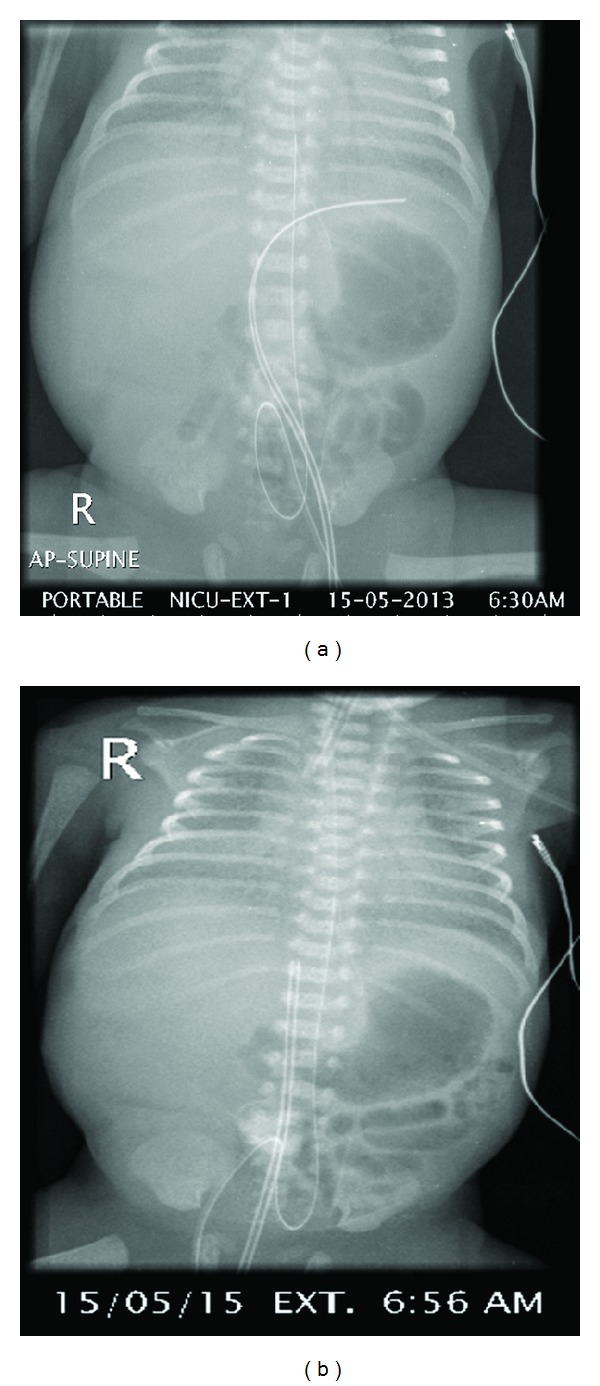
Plain X-ray of the abdomen demonstrating the UVC directed to the left within the splenic vein (a) which is corrected after 26 min (b).
